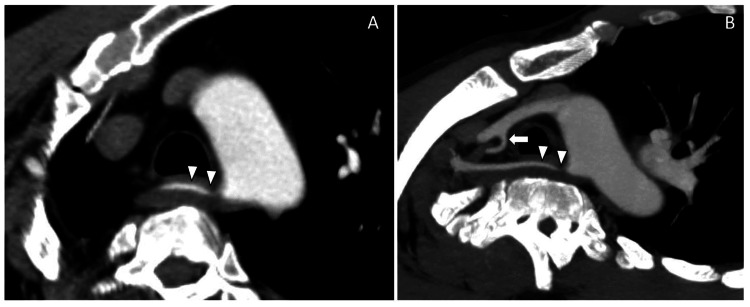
Oblique reformatted thin axial CTA and MIP images showing aberrant RSA origin from the arch of aorta with severe stenosis at the ostio-proximal segment (arrowheads). Note the variant origin of right vertebral artery arising from right common carotid artery (1B, white arrow). CTA: computed tomographic angiography; RSA: right subclavian artery; MIP: maximum intensity projection
